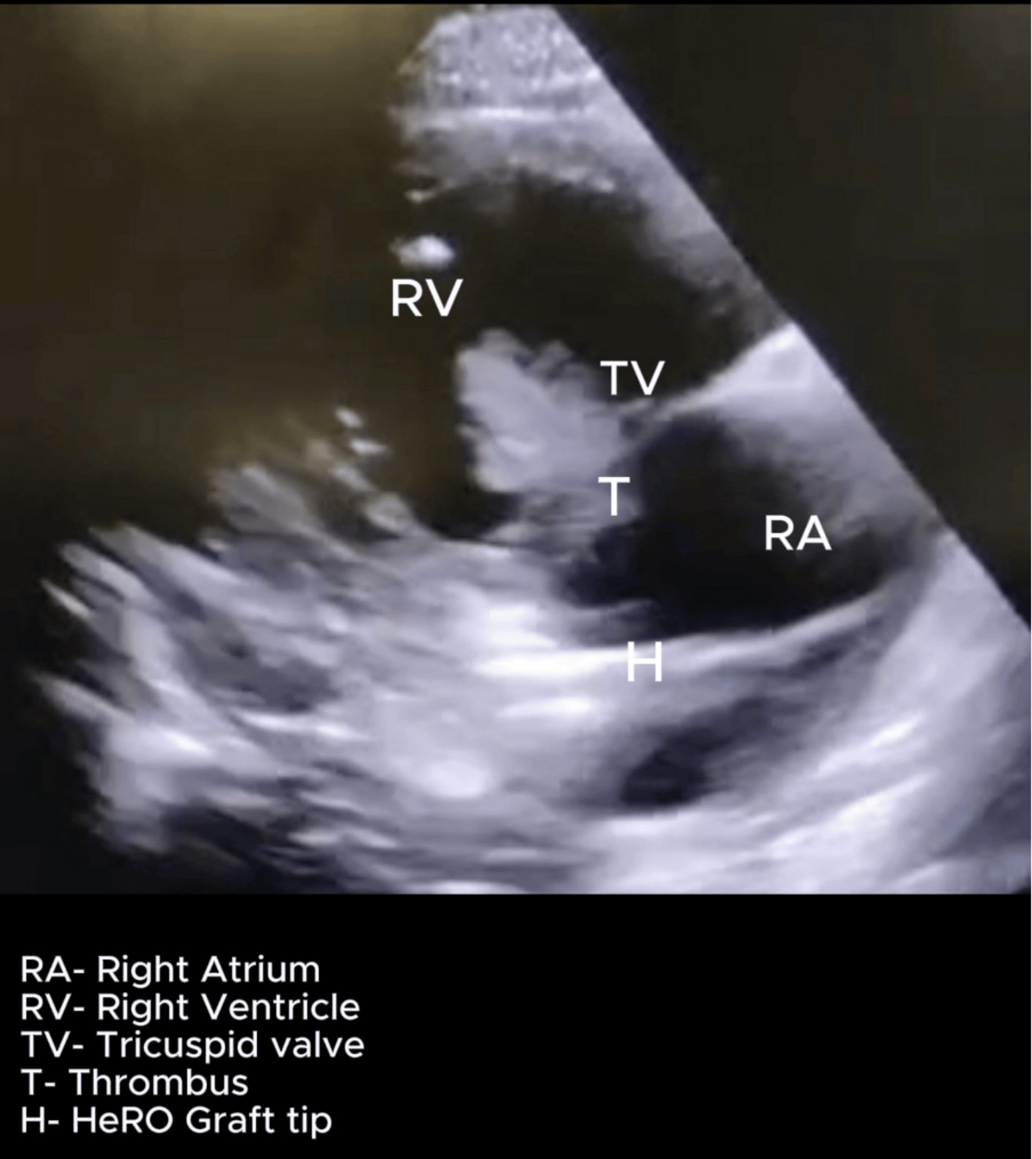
POCUS heart- right ventricle inflow view showing Hero graft tip and thrombus attached to tricuspid valve Probe: Phased array. View: Right ventricle inflow view. Depth: 19 cm POCUS, point-of-care ultrasound; HeRO, Hemodialysis Reliable Outflow
